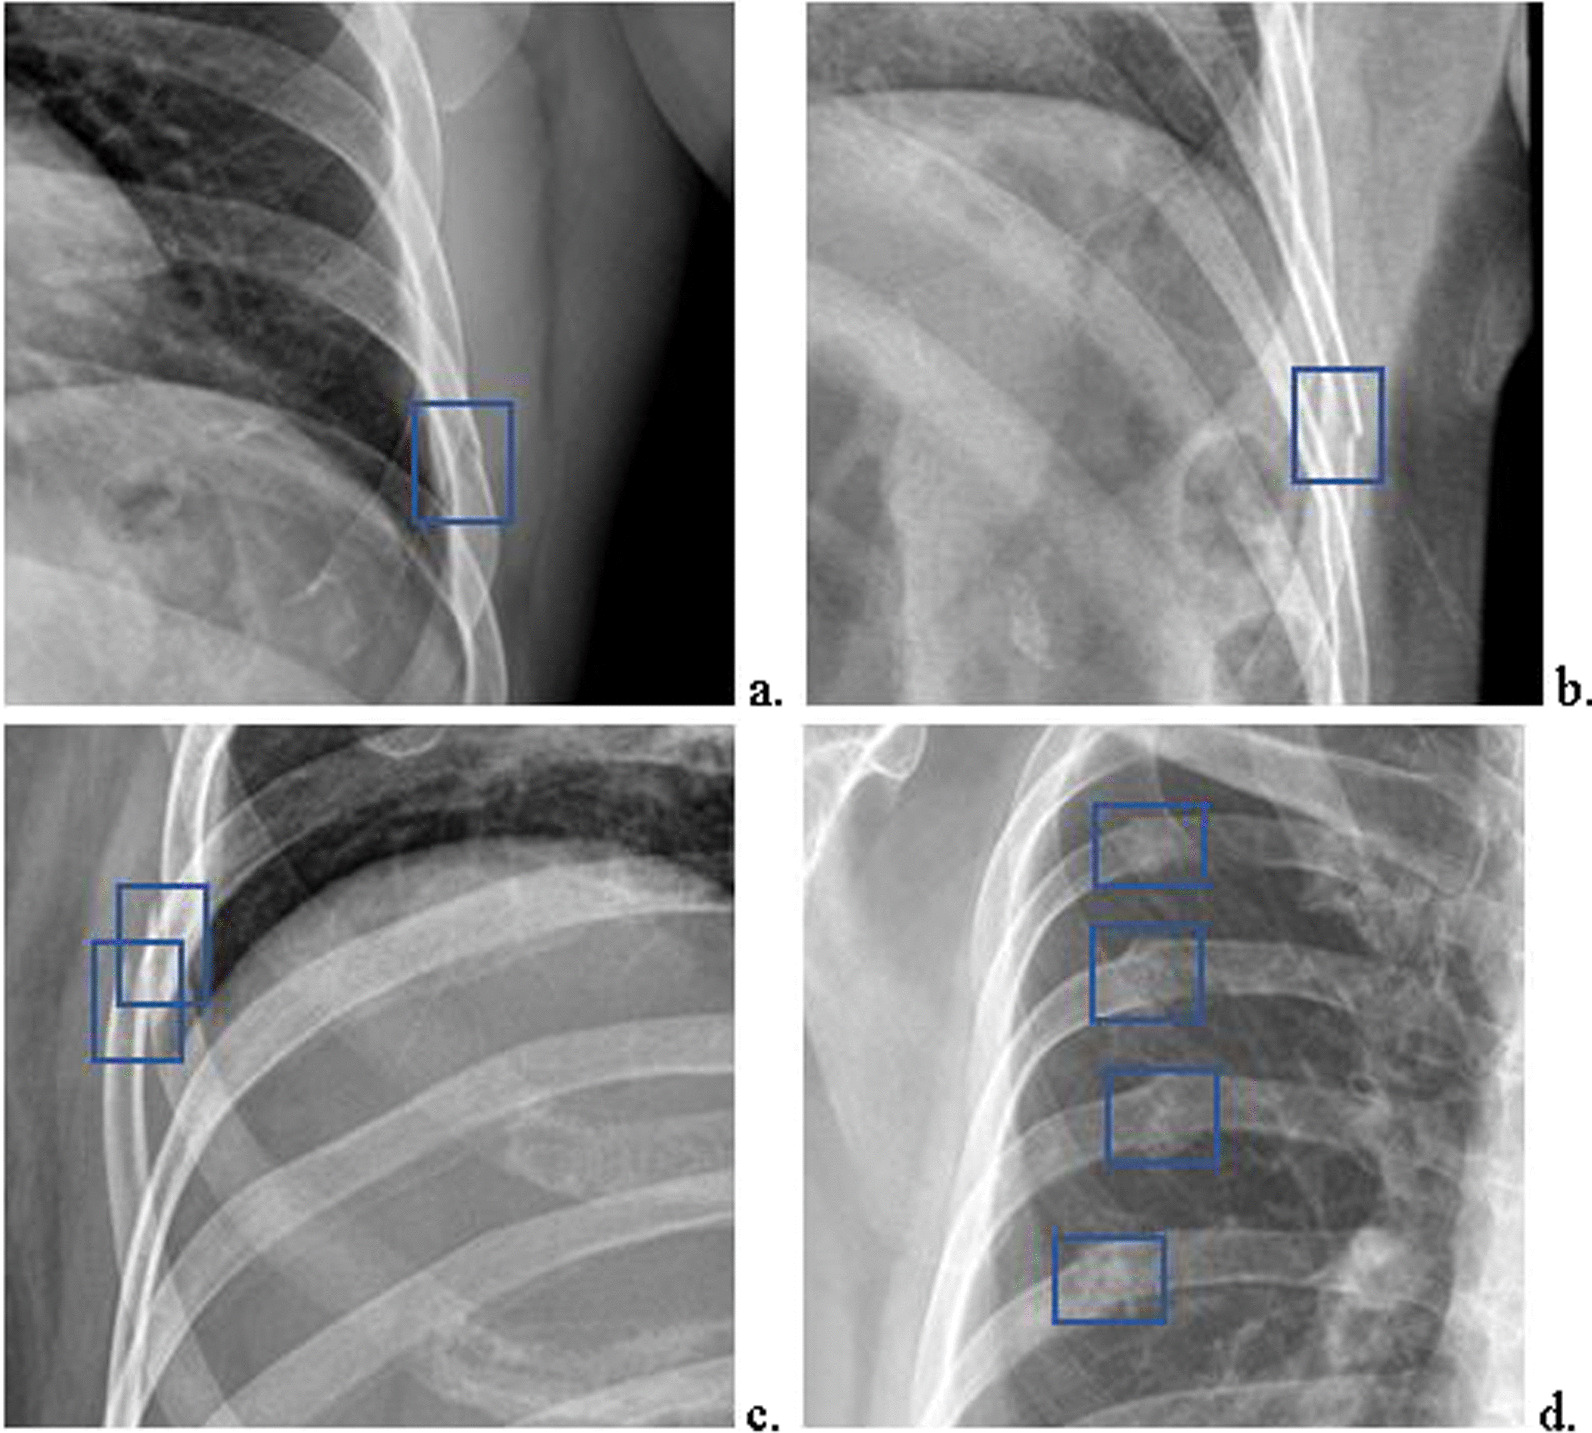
Fracture sites in 1080 radiographs with the following signs were marked by the radiologists. a Complete rip disruption with a lucent line. b Disruption of the inner or outer cortex. c Fracture rib end displaced. d Rib deformity with callus formation
